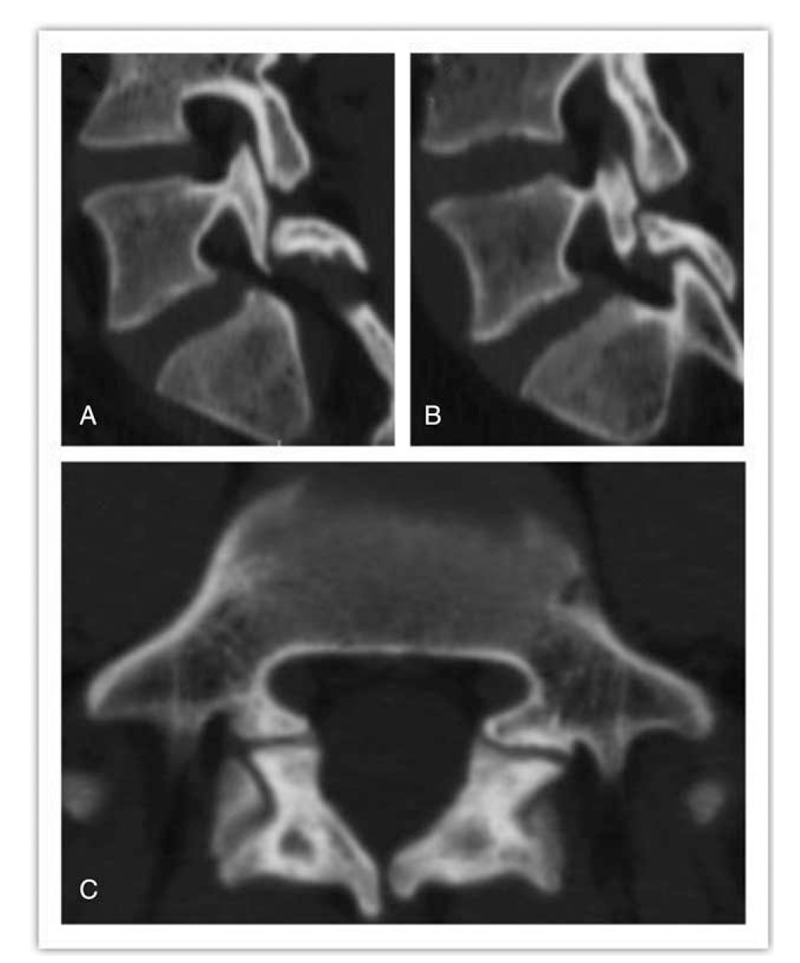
Axial and sagittal computed tomography images showing the presence of bilateral spondylolysis at the L5 level.
